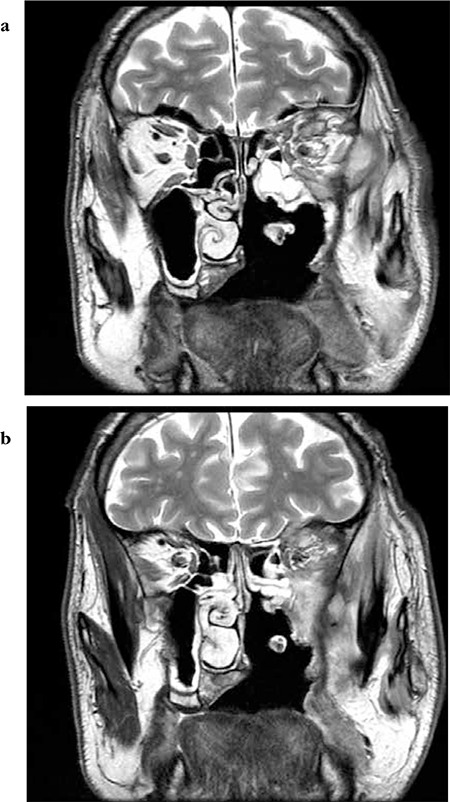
(a and b) Case 3, coronal magnetic resonance imaging images of the orbit showing increased signal intensity of both intraconal and extraconal fat, edematous thickening of the recti and orbital apex infiltrationa
